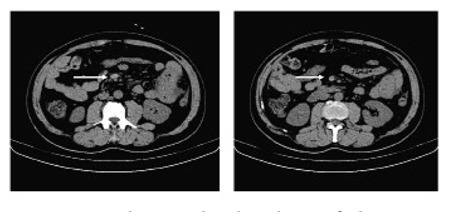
CT showing the thrombosis of the superiormesenteric vein (arrow).
